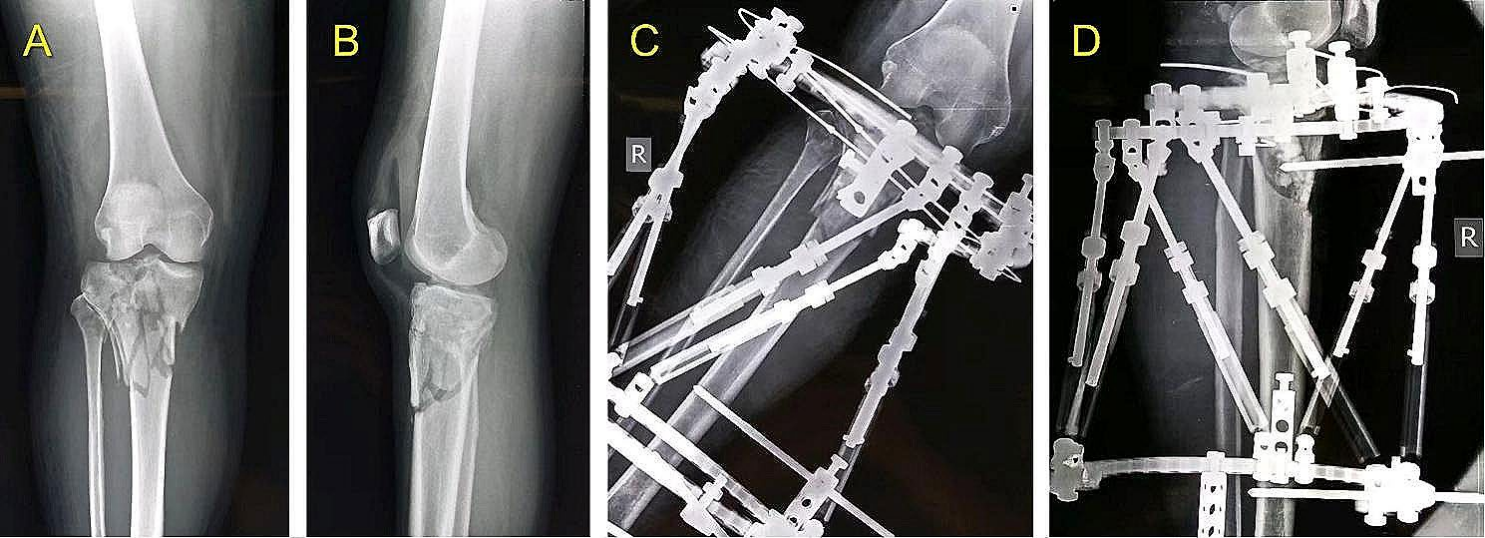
A 47-year-old female who suffered tibial fracture in right leg and treated by the TSF external fixator. (A, B) Immediate AP and lateral views of X-rays after injury. (C, D) Immediate AP and lateral views of X-rays after surgery
